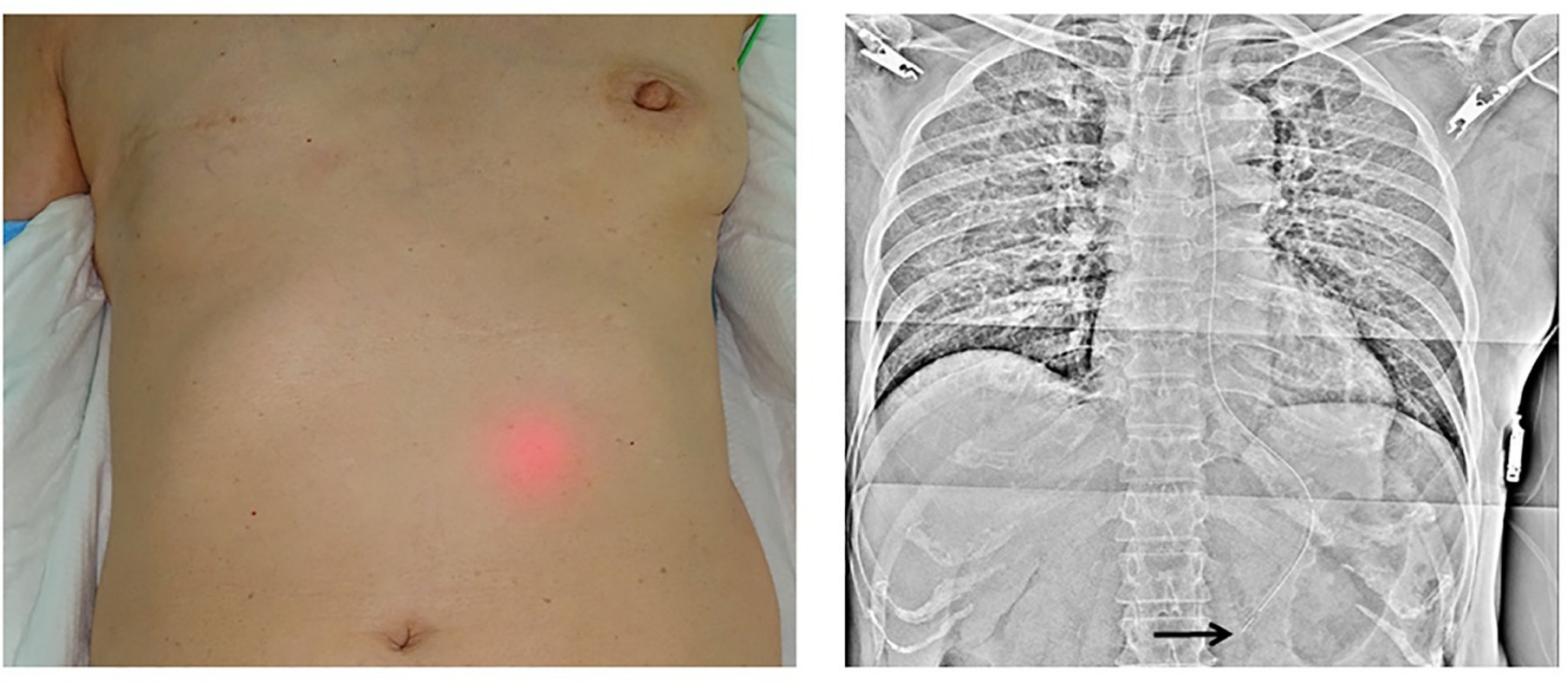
Positive biologically transparent light sign in the mid-epigastric area and corresponding X-ray image. The arrow indicates the tip of the nasogastric tube.
